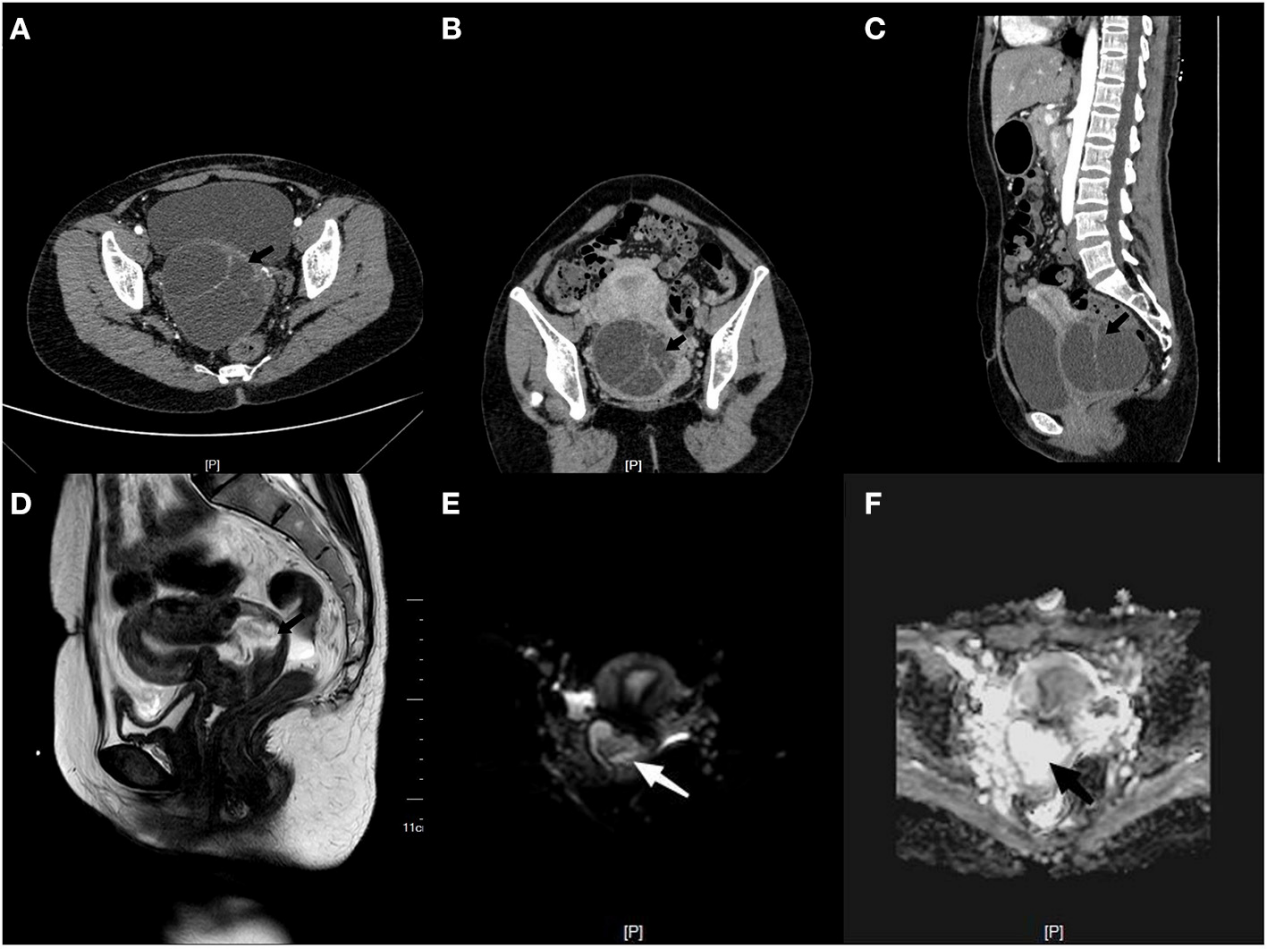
Computed tomography showing the irregular shape of uterus. (A) There is a large abnormal density of multilocular cystic shadow, which is ~8.8 × 8.5 cm in size (black arrow). (B) There is an irregular separation in the cyst, and there is a close relationship between the uterus, cervix, and right appendix (black arrow). (C) Three-dimensional computed tomography reconstruction showing the cyst from below the posterior wall of the uterus to the posterior lip of the cervix (black arrow). (D) After cyst resection, additional MR examination showed irregular shape of posterior lip of cervix, size 4.1 × 2.3 × 4.7 cm, inhomogeneous low signal on T1WI (black arrow). (E) Postoperative lesions under diffusion weighted imaging (white arrow). (F) Postoperative lesions in ADC images (black arrow).
